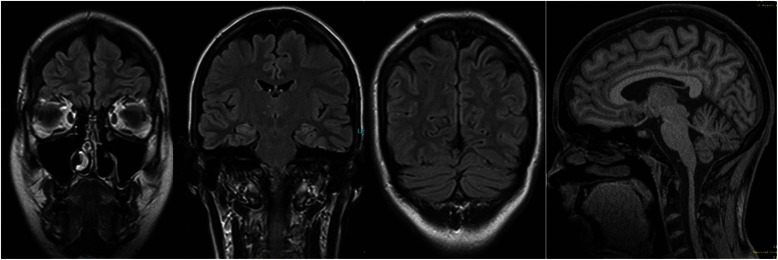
Magnetic resonance imaging shows a slight but atypical age-related frontoparietal and cerebellar atrophy.
